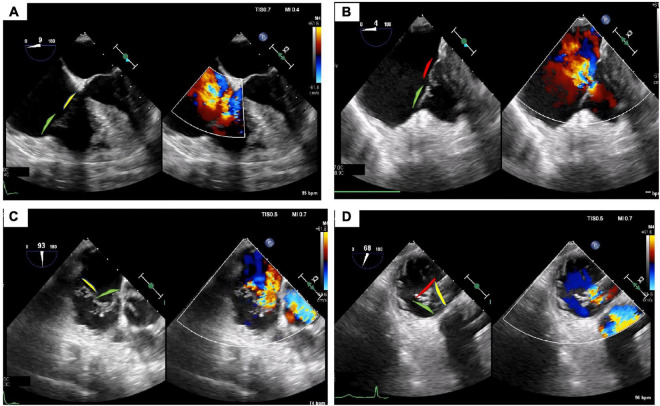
Tricuspid regurgitation treated with 3 TriClips implantation. Transesophageal evaluation of the tricuspid regurgitation (2D/color). (A) 2D mid-esophageal view at 0° showing the anterior and septal leaflet. (B) Deep-esophageal view 0° showing the jet between anterior and posterior leaflet. (C) Mid-esophageal view at 90° showing the jet between the anterior and septal; (D) transgastric view short axis, in systole, the valve is closed, and the jet is mainly located between the anterior and septal leaflet. Anterior leaflet: green; Septal leaflet: yellow; Posterior leaflet: red.
